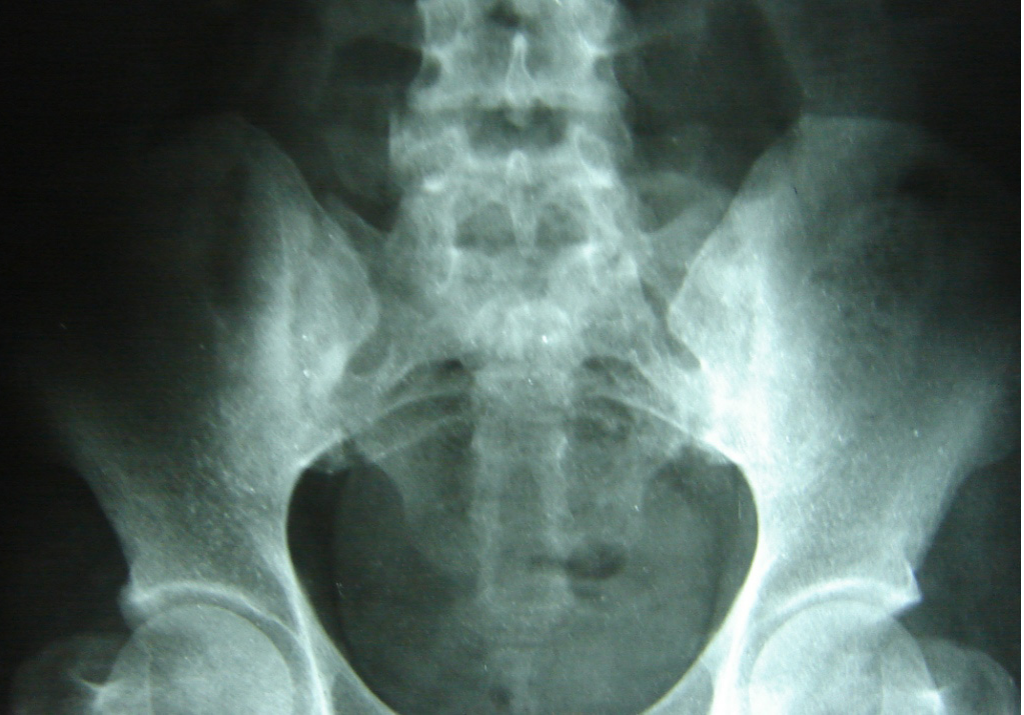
X-Ray of pelvis showing sacroilitis.
